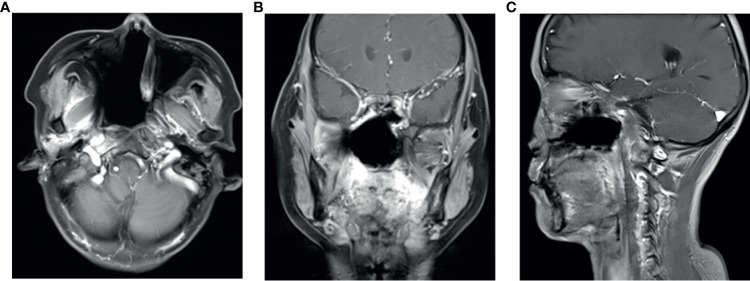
After three cycles of anlotinib treatment, the August 2019 contrast enhancement T1-weighted MR imaging showed the primary tumor was significantly reduced (A–C).
